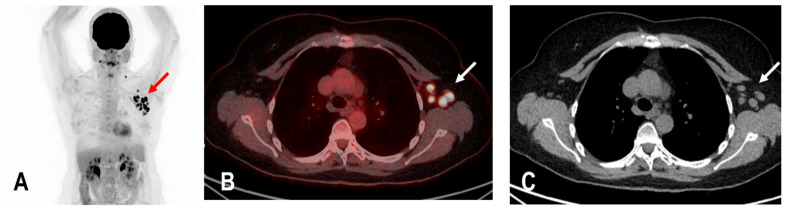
The maximum intensity projection (MIP) (A) of a patient with rectal carcinoma and HLA in the ipsilateral draining supraclavicular and axillary lymph nodes (red arrow) in the ^18^F-FDG PET/CT 10 days following COVID-19 vaccination. Fused images (B) show that the hypermetabolism is located in the moderately enlarged lymph nodes (white arrow) which still show a physiological fat hilus (SUVmax: 18.6, rHLA/MBP: 7.75, and rHLA/RL:11.6 (C)).
